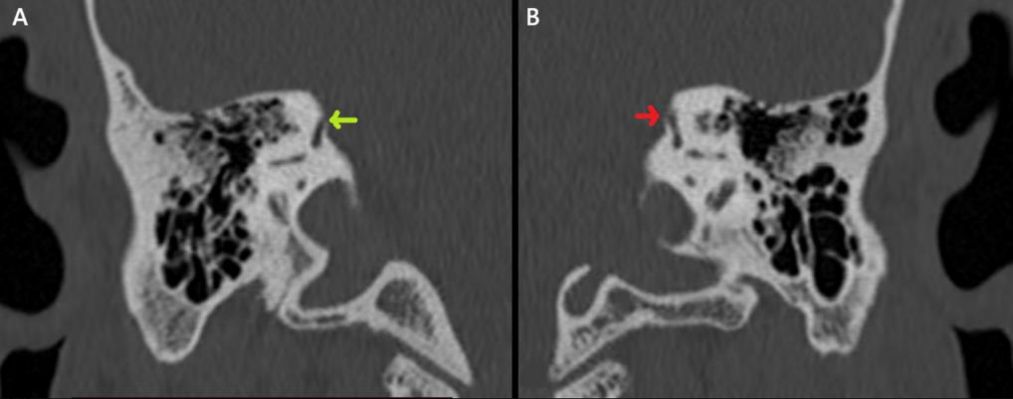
frontal view of the patient´s temporal bone CT scan showing a dehiscence of the right superior semicircular canal (A); and (B) no bone defect of the left superior semicircular canal
